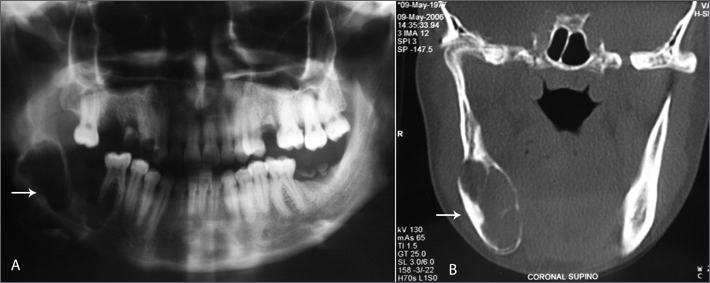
Lytic insuflating lesion with regular borders and fine internal septations, located in the right mandibular body. (A) panoramic mandibular radiograph (B) computed tomography.
